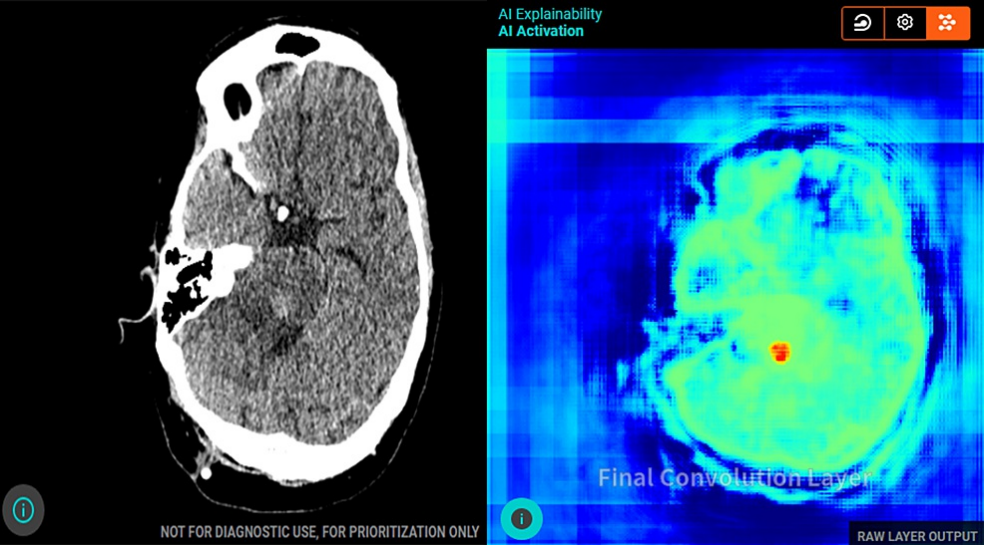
Axial non-contrast CT of the brain with AI tool analysis. (a) Shows a hyperdense area in the posterior pons that is concerning for an acute intracerebral hemorrhage as per AI analysis. (b) Shows the final convolution layer and feature map that the AI tool uses to identify the ICH. AI, artificial intelligence; ICH, intracranial hemorrhage.
